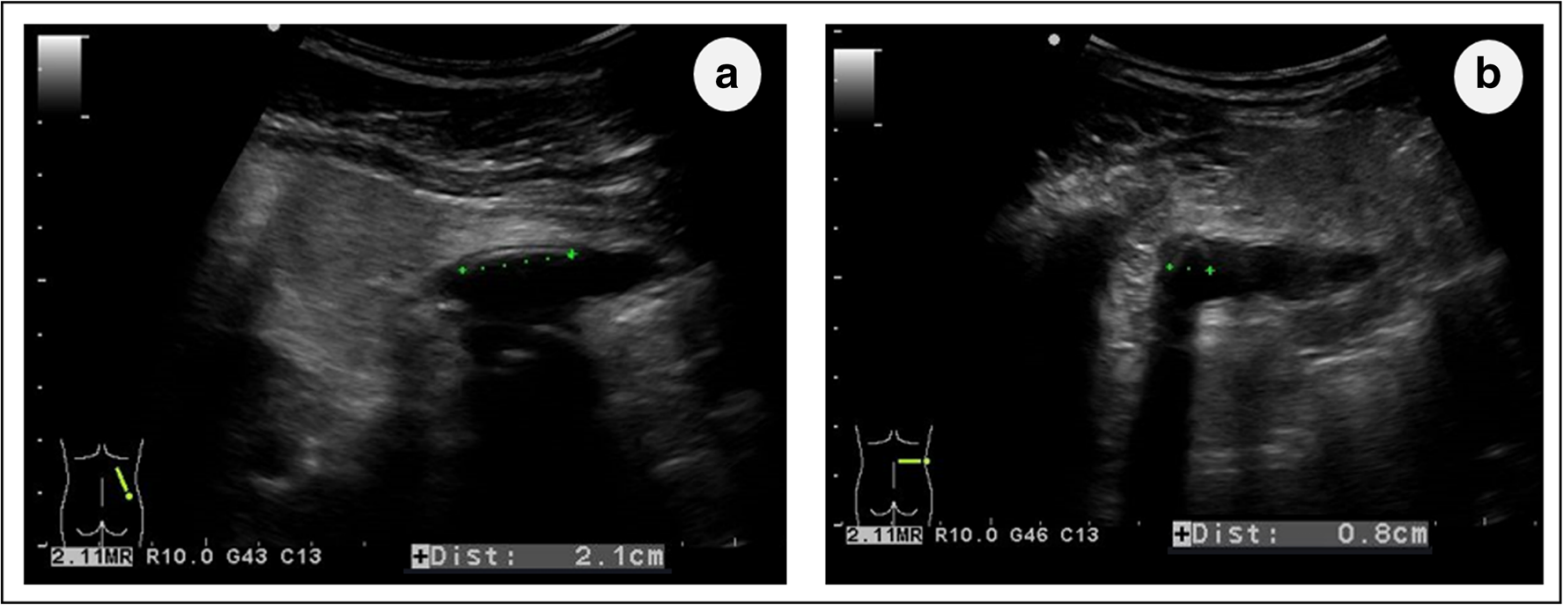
Longitudinal (a) and transverse (b) ultrasound picture of the structure in the renal pelvis
